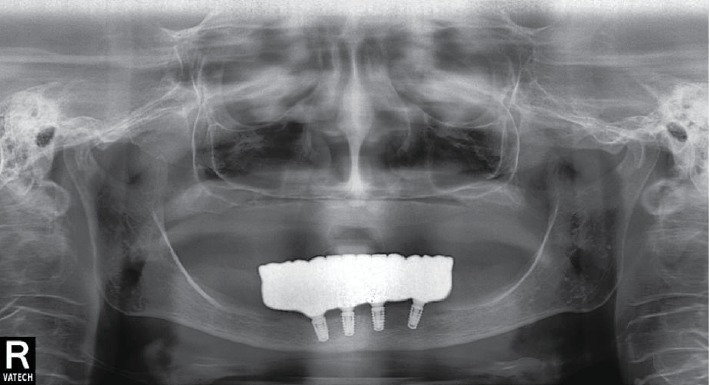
Panoramic view. Radiographic follow-up a year after surgery.
